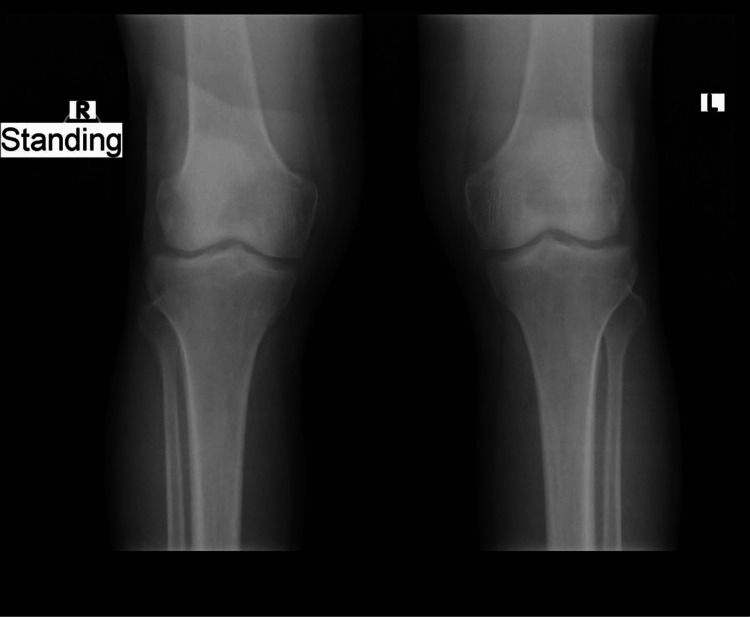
Knee radiograph showing normal articulation of bones forming the knee joint.
